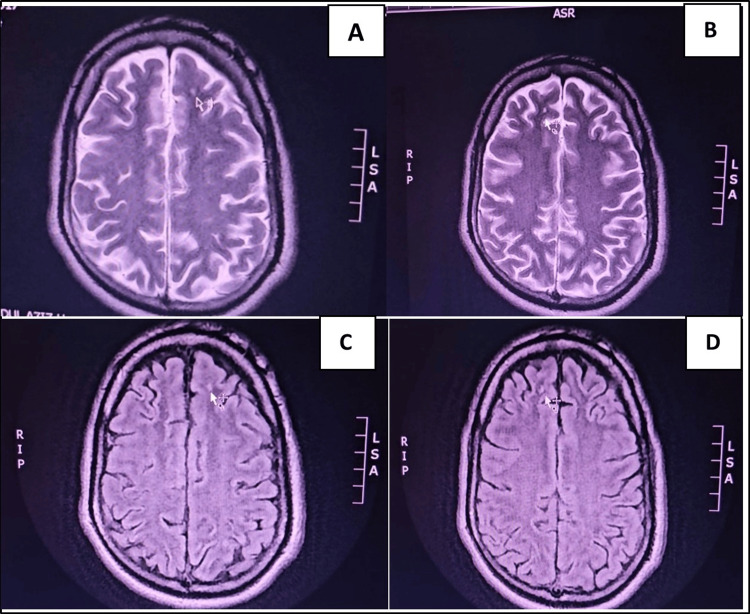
Axial brain MRI images showing frontal changes Axial brain MRI images; T2-weighted. A, B and axial FLAIR C, D, showing Rt. and Lt. frontal subcortical small hyperintense areas (arrows).
